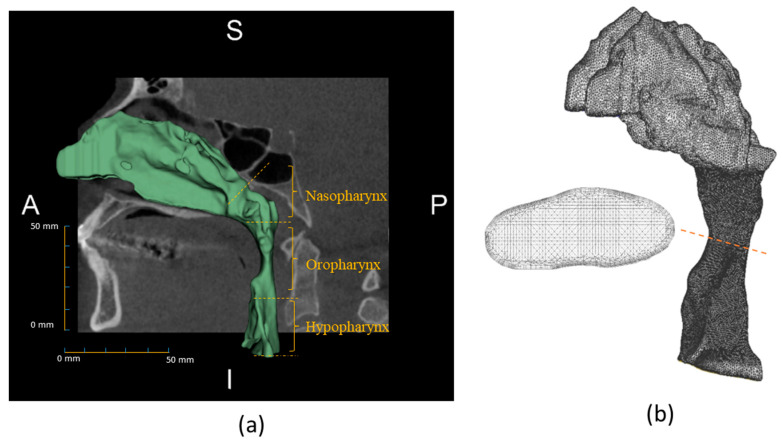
(a) Extracted airway geometry from cone-beam CT images with specified pharyngeal sections. (b) The meshed volume of the airway with a cross-sectional view at the location is denoted by the dashed line.
